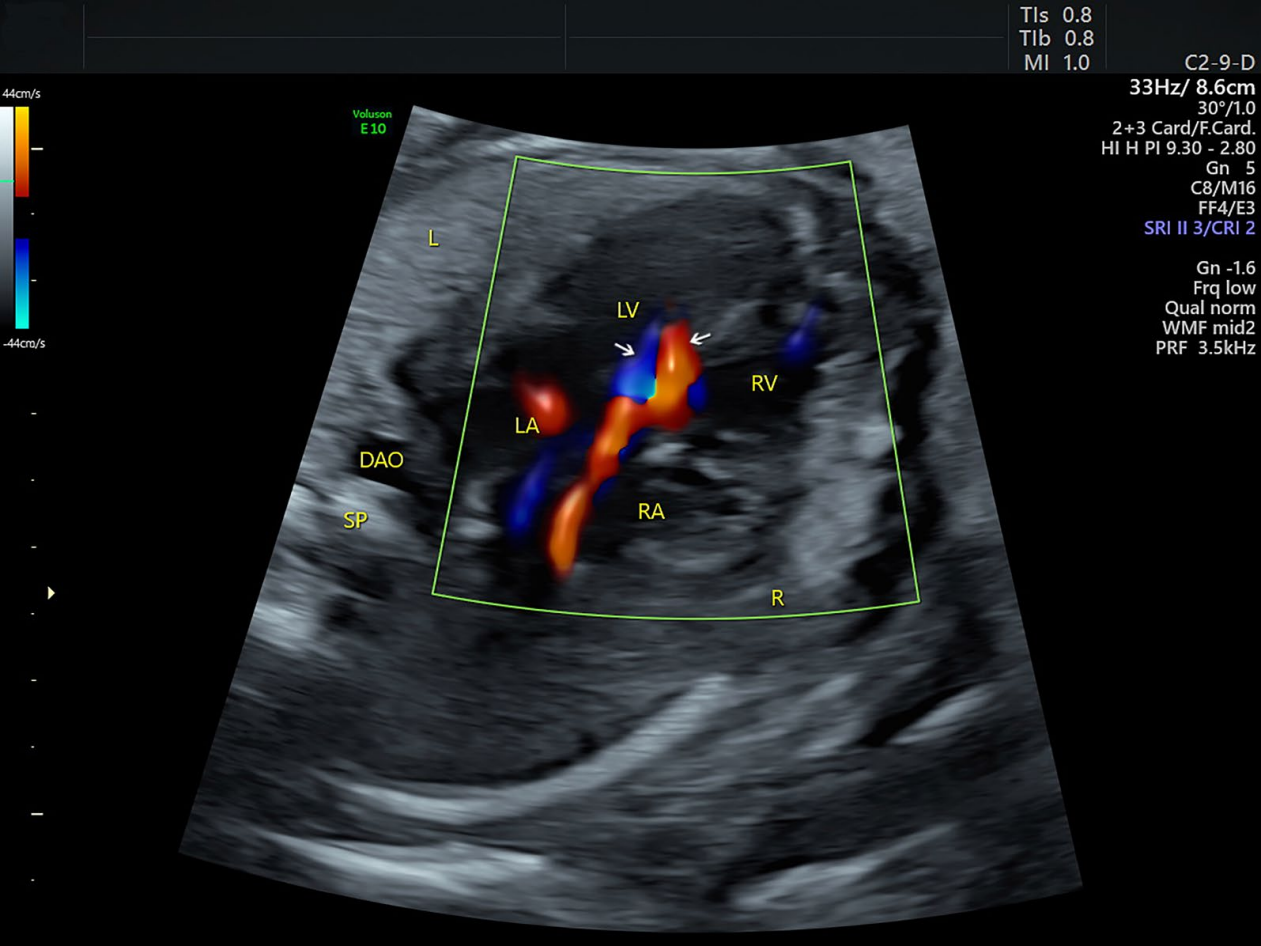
Sonograms of the fetus of coarctation of the aorta at the four-chamber view. The four-chamber view shows symmetrical left and right chambers. In addition, a bidirectional shunt (indicated by the arrows) between the left and right ventricles is demonstrated by color Doppler. DA: ductus arteriosus; DAO: descending aorta; L: left; LA: left atrium; LV: left ventricle; R: right; RA: right atrium; RV: right ventricle; SP: spine
